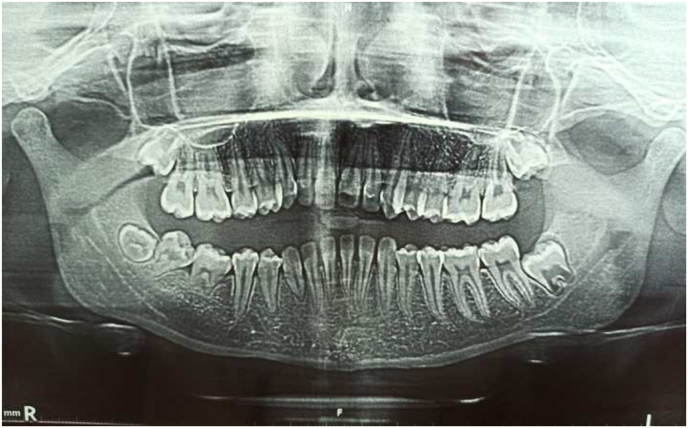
Panoramic radiography showing the shortened root of 47/48 and an abnormal development of 48.
